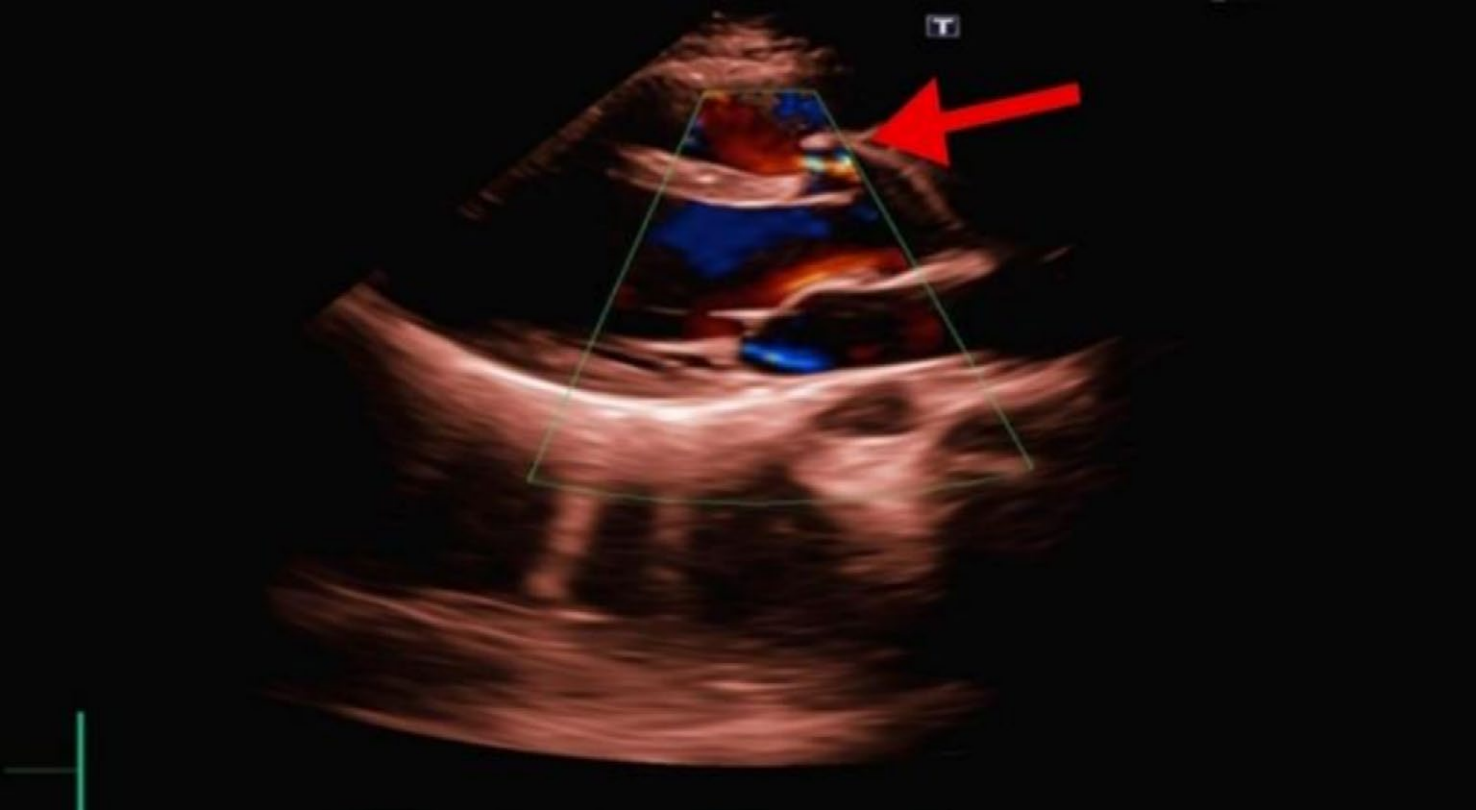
TTE parasternal long‐axis view showing right sinus of Valsalva rupture into right ventricle.
